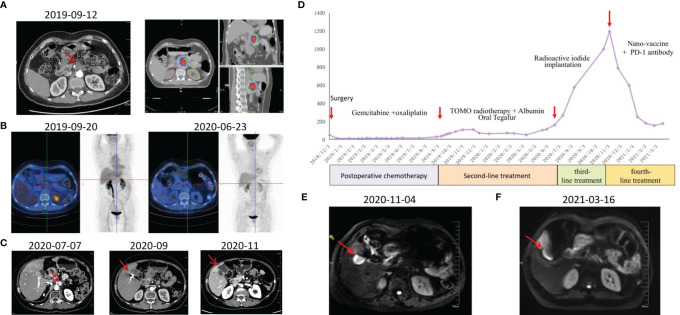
Examinations of patient in different periods. (A) CT scans revealed retroperitoneal lymph node metastasis 9 months after surgery and tomotherapy was performed. (B) PET-CT scans were performed before and approximately 9 months after treatment. (C) CT scans during third-line treatment. (D) CA19-9 levels of this patient throughout the treatment. (E) MRI scan was performed before immunotherapy. (F) MRI scan was performed approximately 4.5 months after immunotherapy.
